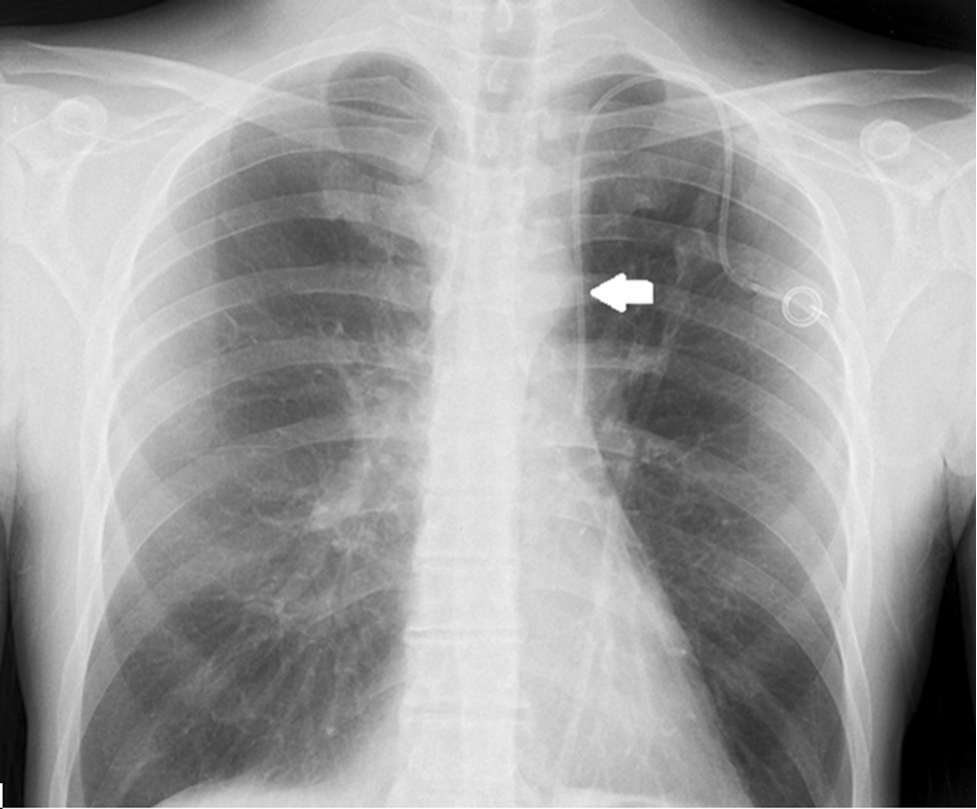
Frontal chest X-ray showing a portacath with left jugular vein approach and demonstrating the unusual course of the catheter in the left hemithorax (arrow), rather than in the right normal anatomical side of the superior vena cava.
